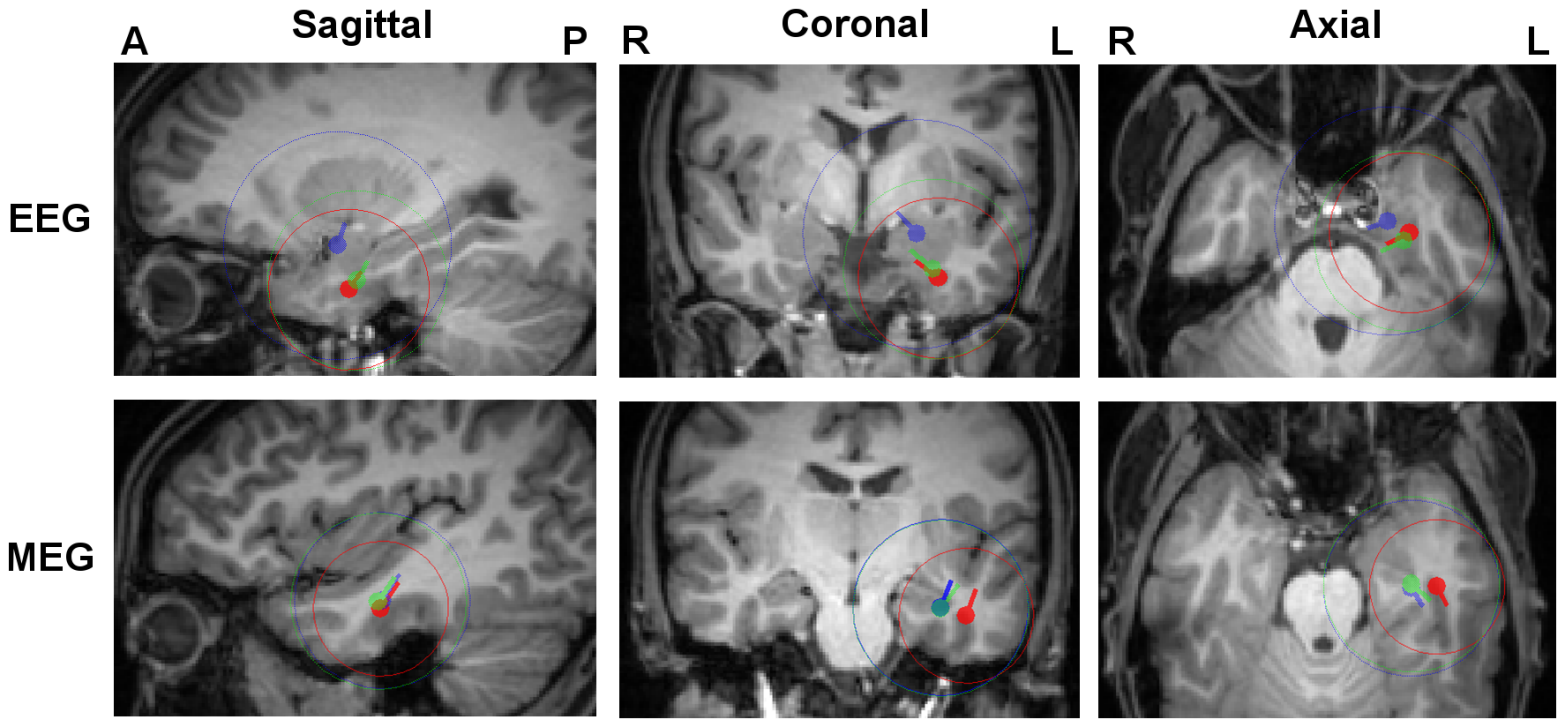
Comparison of 3 and 6 compartment head models. FT9 centroids and spread spheres plotted on T1w-MRI for head models *6C_Cal* (red), *3C_Cal* (green) and *3C_100* (blue). The centroid locations of *6C_Cal* were used for the selection of MRI slices and all results were projected on these slices.
